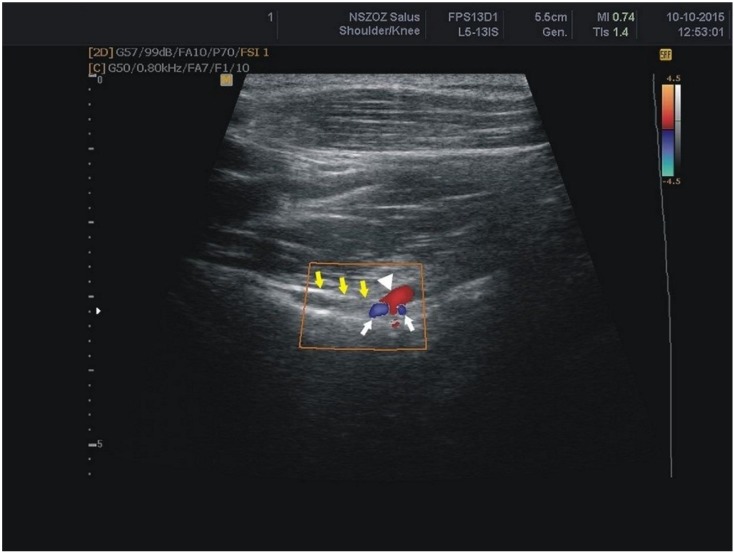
Sonogram of the suprascapular notch region (color Doppler) white arrows: suprascapular veins; arrowhead: suprascapular artery; yellow arrows: superior transverse scapular ligament.
